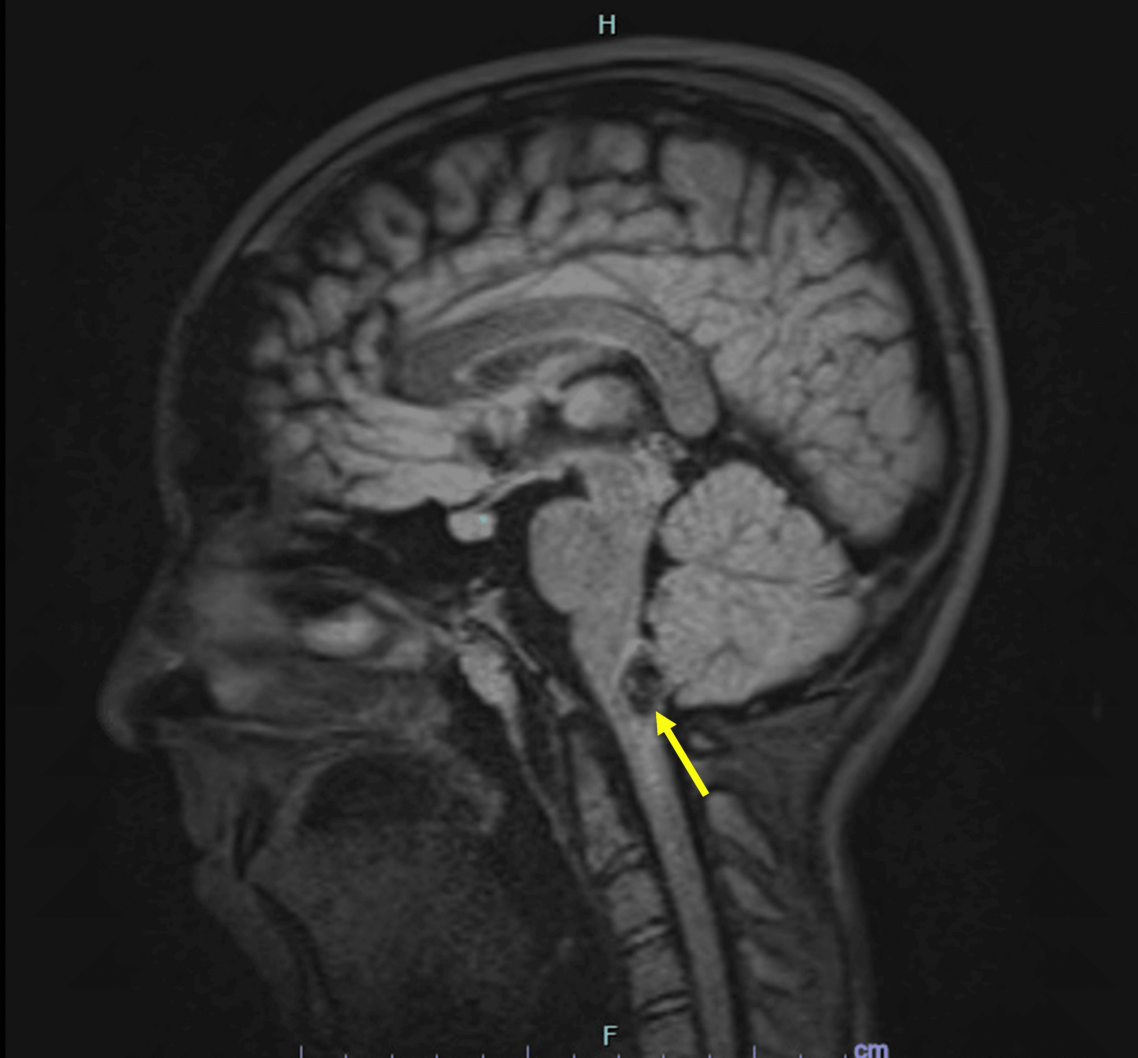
Sagittal T1-weighted MRI demonstrating a nodular intra-axial lesion of the dorsal medulla with intrinsic hyperintensity consistent with subacute hemorrhage (arrow), suggestive of cavernous malformation. MRI: Magnetic resonance imaging
